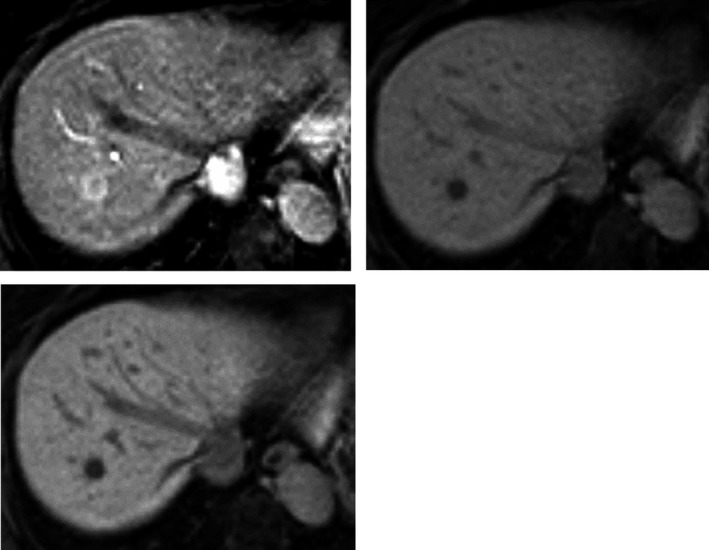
On gadoxetic acid (Gd‐EOB‐DTPA)‐enhanced magnetic resonance imaging (MRI), abbreviated as EOB‐MRI, the mass was enhanced in the arterial dominant phase and washed out in the late phase and hepatocyte phase
